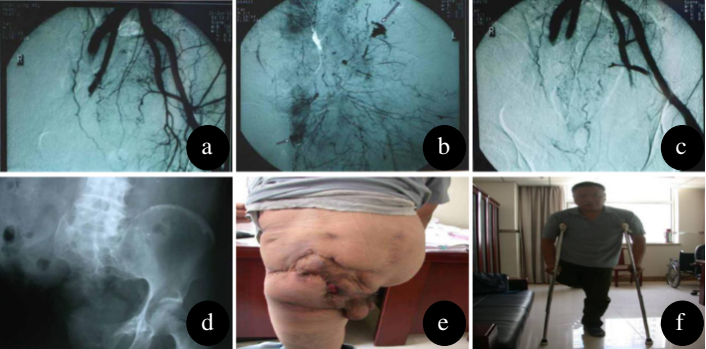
Male, 45 years old, admitted for right hemipelvectomy, 12 h after he was involved in a traffic accident. (a) The preoperative angiography showed that the right internal and external arteries were occluded, and the left ones were normal. Hemorrhage from the small arteries was observed. (b) The magnified picture in some portions shows active hemorrhage from the small arteries. (c) After angiographic embolization of the left internal iliac artery and right internal and external arteries, no hemorrhage was observed. (d), (e), (f) Radiography of the pelvis seven years after the procedure, the wound was healed, mild anal mucosal prolapse was noticed, the patient could ambulate with crutches.
